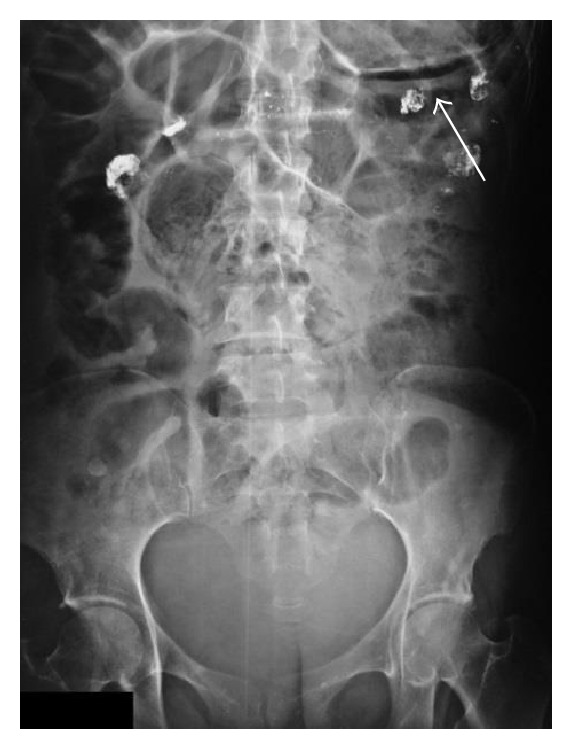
Plain abdominal X-ray. Radiolucent image in the upper left abdominal quadrant, showing the presence of air within the wall of the stomach (arrow).
